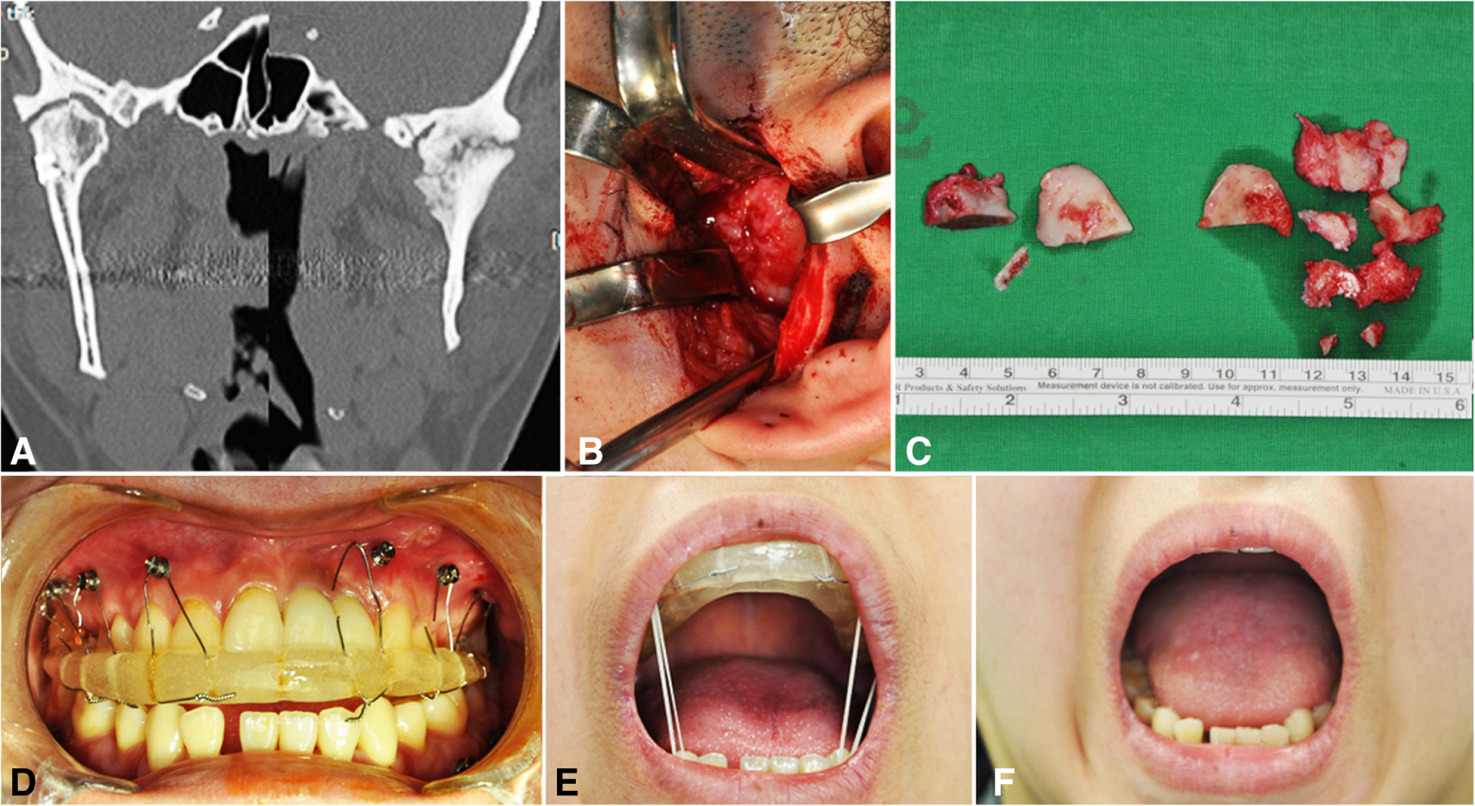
A 23-year-old female, bilateral TMJ ankylosis secondary to trauma, showing coronal CT scan (a), endaural approach with ankylosed mass resection at the left condyle (b), resected coronoid process and ankylosed bony mass from both sides (c), interocclusal splint retained on the maxillary screws (d), active mouth opening exercise with maximal interincisal opening (e), and mouth opening appearance after 6 years (f)
